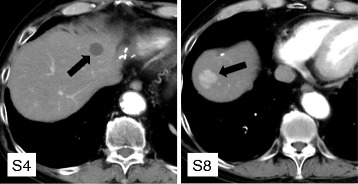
CT appearance of two liver tumors, in segments 4 and 8
